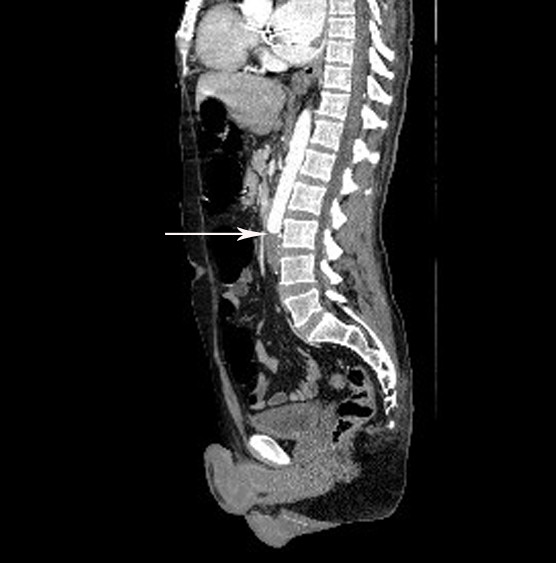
Sagittal CT section showing a thrombus in the distal aorta (arrow).
